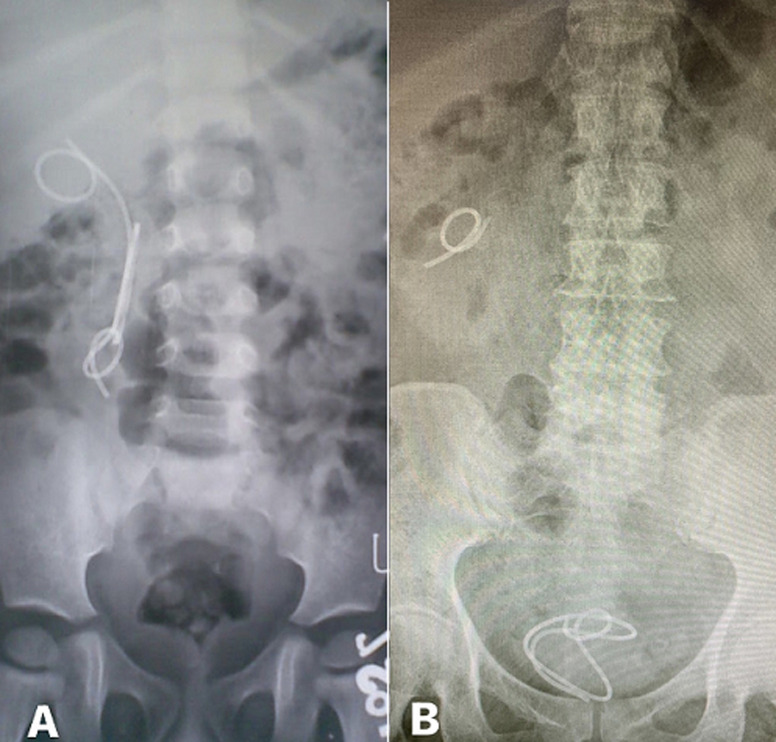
A) plain radiograph showing proximal migration of a retained ureteral stent; the stent was removed using ureterorenoscope; B) a plain radiograph showing fragmented retained ureteral stent with migration of lower end into the bladder which was removed cystoscopically; the proximal end was removed using a ureterorenoscope
